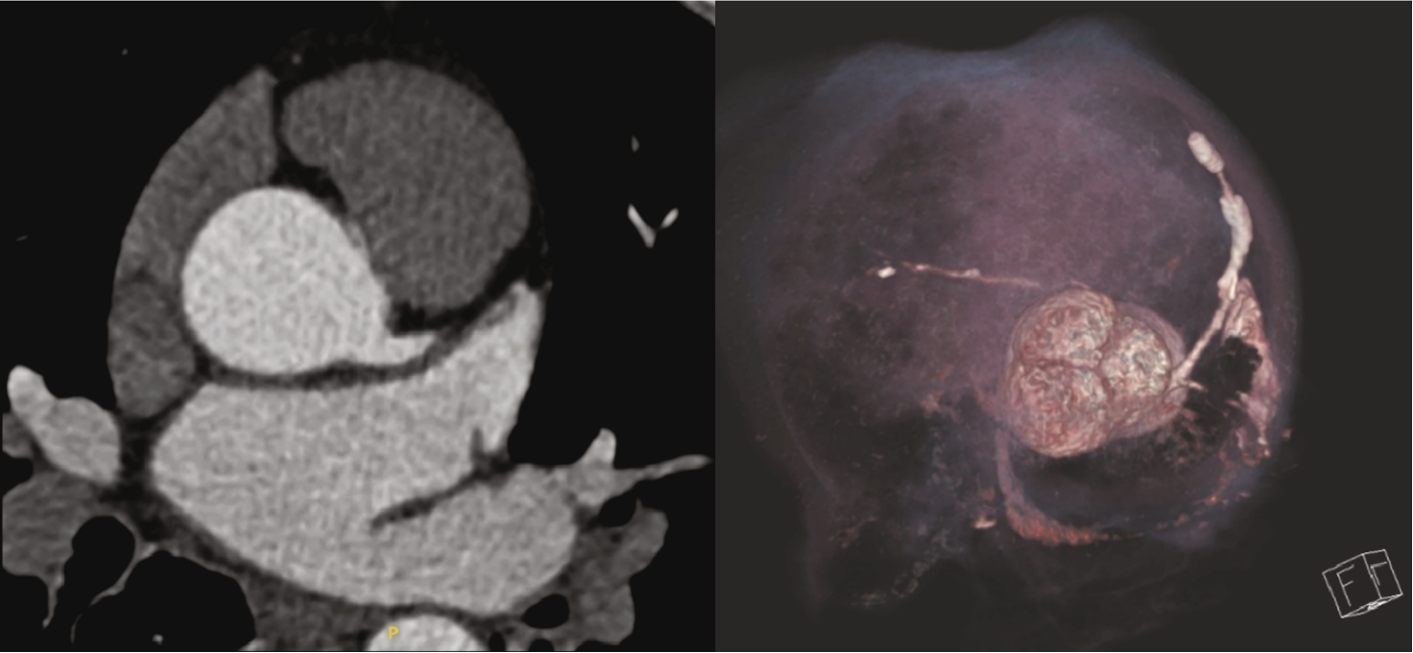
CT coronary angiogram showed anomalous origin of the RCA from the left coronary cusp, with a malignant course between the aorta and pulmonary artery.
